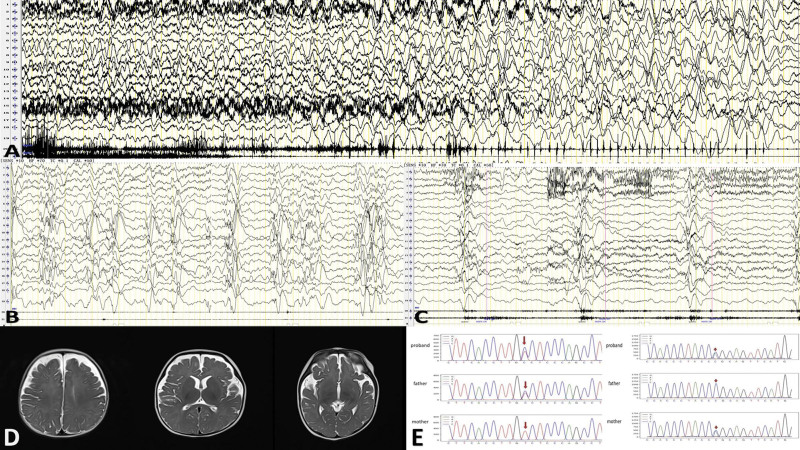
Case 3, male. (A) the “slow” patterns with BHS at 4 mo age. (B and C) VEEG at 8 mo age: B showed hypothalamia (significant at the occiput); (C) 3 times spasms. (D) MRI showed Bilateral front and temporary extra brain spaces slightly increased, (E) LCT gene c.4583A > G, c.4867-5C > A heterozygous mutation. BHS = breath-holding spells, MRI = magnetic resonance imaging, VEEG = video electroencephalogram.
